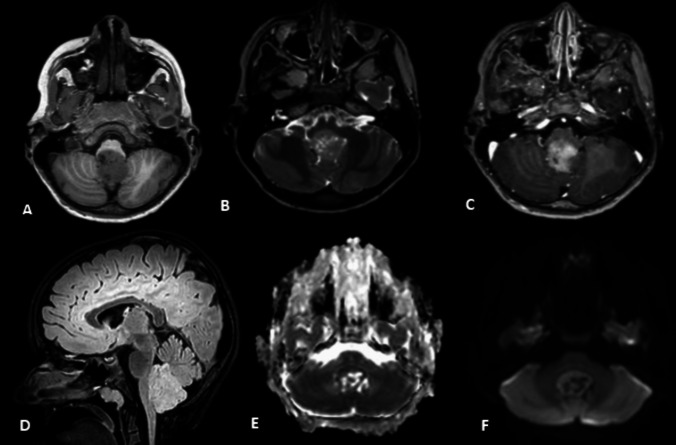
The preoperative MRI findings. A T1-weighted axial image shows a heterogeneous hypointense lesion with contact to the brainstem. B T2-weighted axial image shows an intermediate heterogeneous lesion with minimal perifocal edema and small cystic components. C Axial contrast-MRI with inhomogeneous moderate enhancement of the lesion. D Sagittal FLAIR sequence that demonstrates the cranio-caudal extension of the tumor and shows a minimal increase of FLAIR signal intensity. E, F Apparent diffusion coefficient (ADC) imaging and diffusion-weighted imaging show partly moderate restricted diffusion with multiple small cystic components with proportionately small areas with significant cerebral blood volume increase
